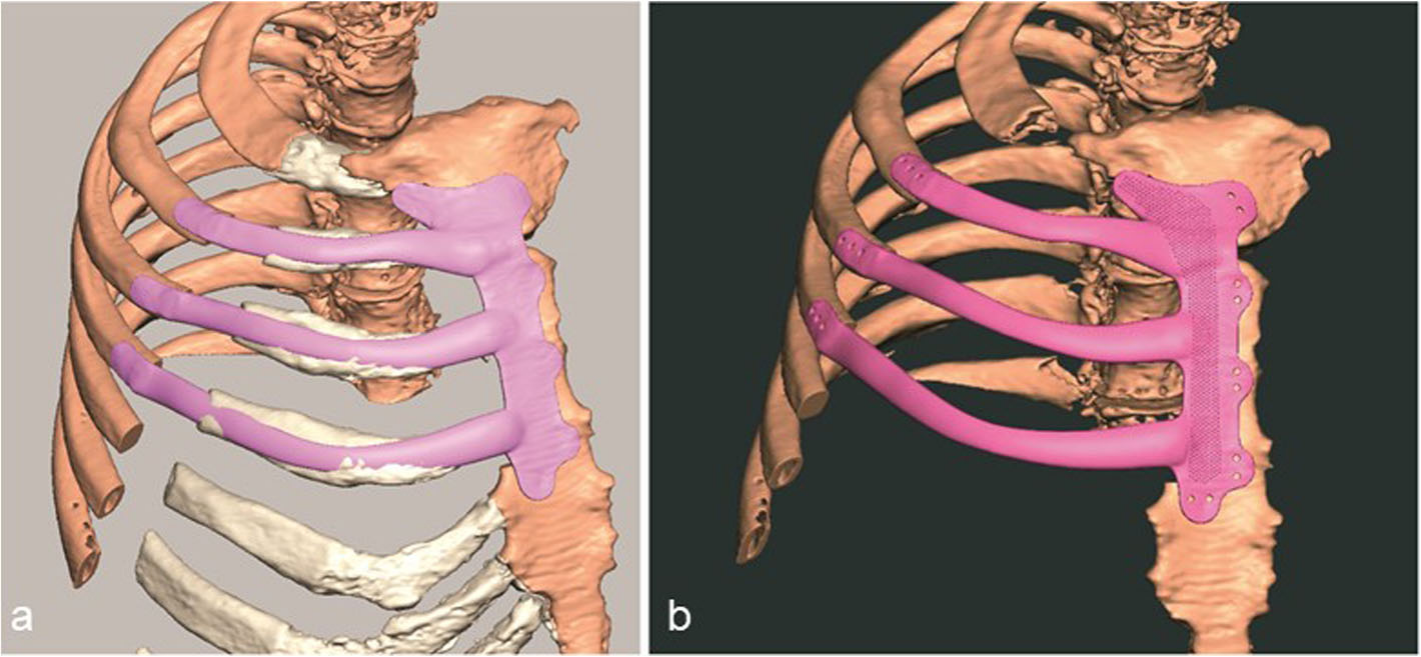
An anatomical image of the ribs and right hemi-sternum obtained digitally from the CT scan (a) and the titanium implant fashioned anatomically to mimic their shape and surface contours, namely the depressions and ridges of the sternum and ribs (b)
